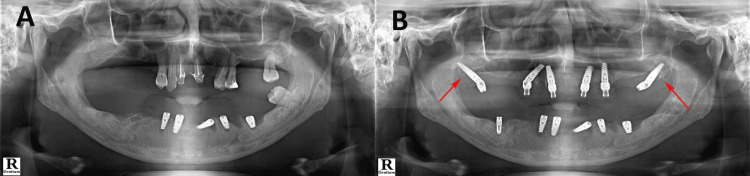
Orthopantomographic (OPG) image (A) Pterygoid region before implant placement, (B) After pterygoid implant placement This OPG image is of a patient from the study and is used with the permission of the patient.
